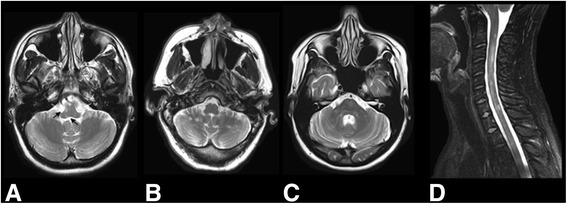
Axial T2-weighted MRI images of the infratentorial region from three unique patients demonstrating foci of T2-hyperintensity at the brainstem (right pontomedullary sulcus and medial vestibular nucleus (arrows)) (a), bilateral cerebellar lobes (b), and brainstem and bilateral cerebellar peduncle regions (c). Sagittal T2-weighted MRI image of the cervical spinal cord revealing multi-focal regions of T2-hyperintensity within the parenchyma (d)
